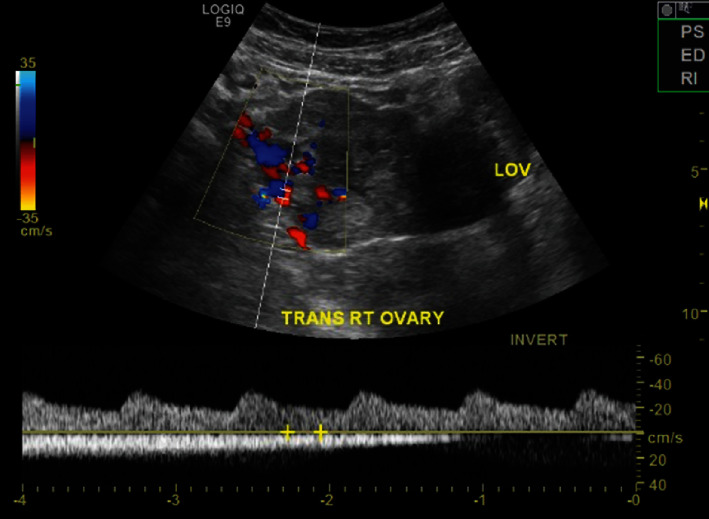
Right adnexa with Doppler flow.
